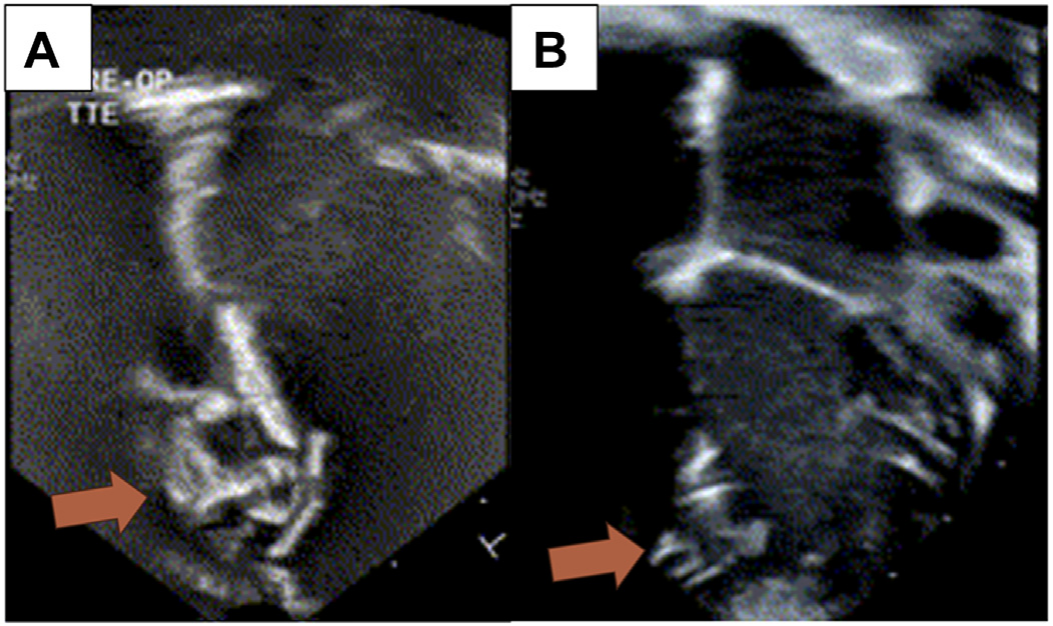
(A) Preoperative and (B) postoperative transthoracic echocardiogram of the apical septal region showing devices and septal defect repair (arrows).
